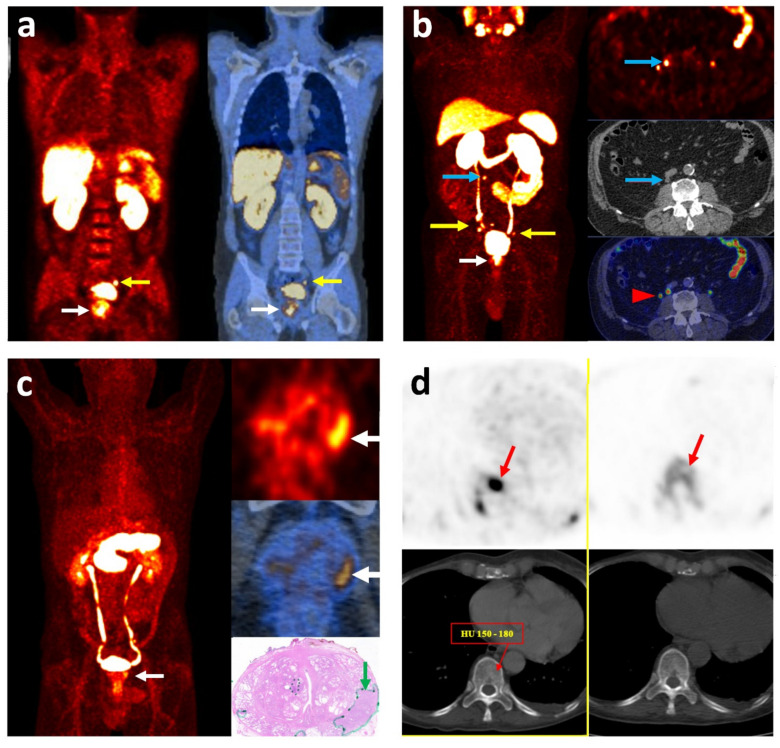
Imaging with different radiotracers localizing primary prostate cancer and its metastasis. (a) [^18^F]choline PET/CT: coronal fused images in a high-risk patient with GS = 8 and PSA = 9.6 ng/mL: primary lesion in the prostate gland (white arrow) and a metastatic lymph node in the left iliac chain (yellow arrow). (b) [^68^Ga]Ga-PSMA PET/CT: MIP (right) and transaxial (left) images in a high-risk patient with GS = 8 and PSA = 4.8 ng/mL: primary lesion in the prostate gland (white arrow) and multiple metastatic lymph nodes in the pelvis (yellow arrow). An unexpected small lymph node is also detected in the upper retroperitoneal region (blue arrow). The red arrowhead shows the physiologic activity in the right ureter. (c) [^68^Ga]Ga-RM2 PET/CT: MIP (right), transaxial (left) images and pathology section (right lower) in a high-risk patient with pT3a N1 (1/23) and GS = 9: primary lesion in the prostate gland (white arrow). The tumor is outlined with a green line and arrow in the pathology section. (d) Transaxial images of [^18^F]choline PET/CT (left) and [^18^F]Na PET/CT (right) in a high-risk patient, showing an early bone marrow metastasis (red arrow) without morphological changes on CT. GS: Gleason Score; MIP: Maximum Intensity Projection; PSA: Prostate-Specific Antigen.
